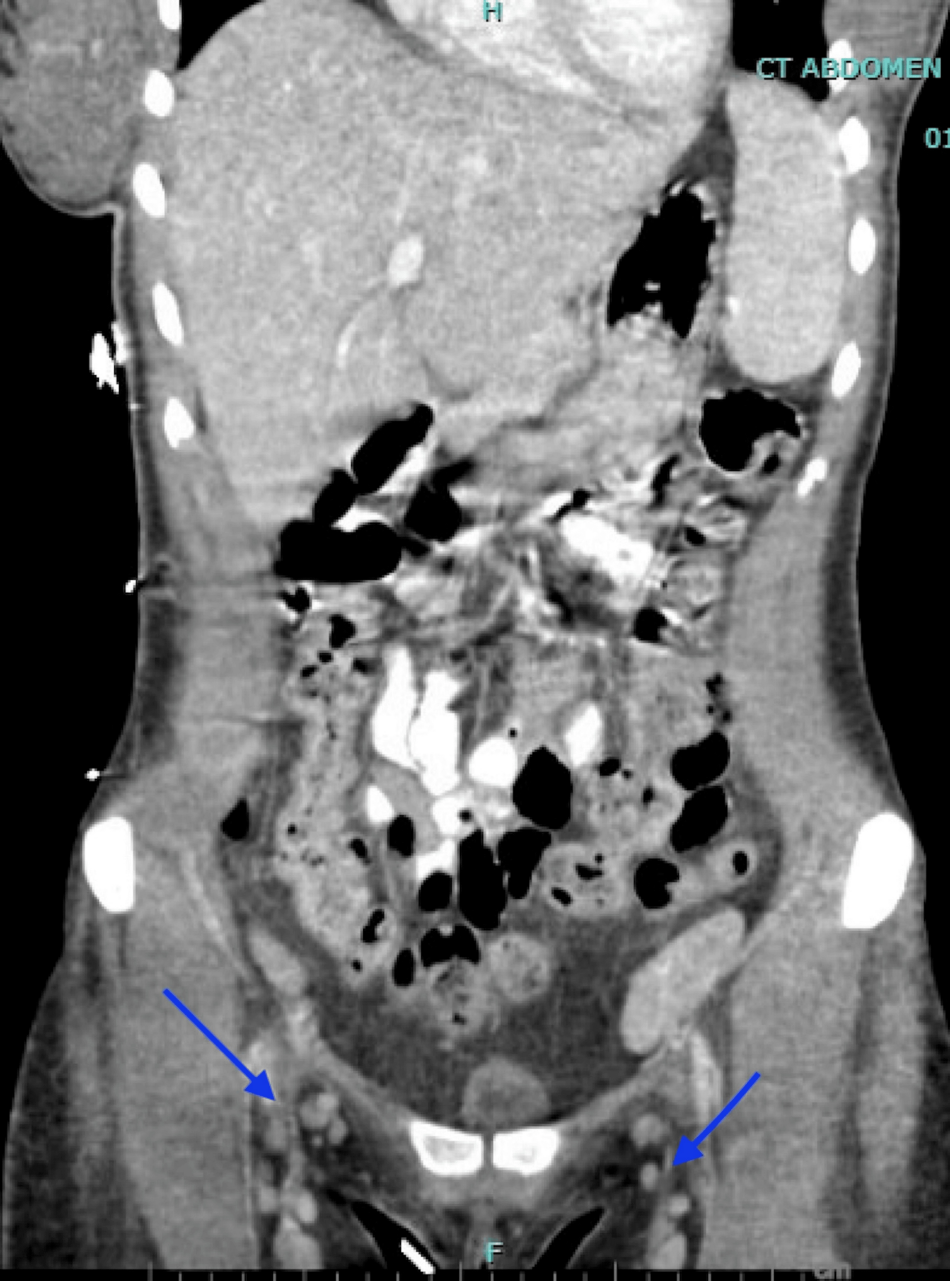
CT Abdomen and Pelvis with Intravenous Contrast in the Coronal Plane Blue arrows show numerous bilateral subcentimeter inguinal lymph nodes. CT: Computed Tomography
